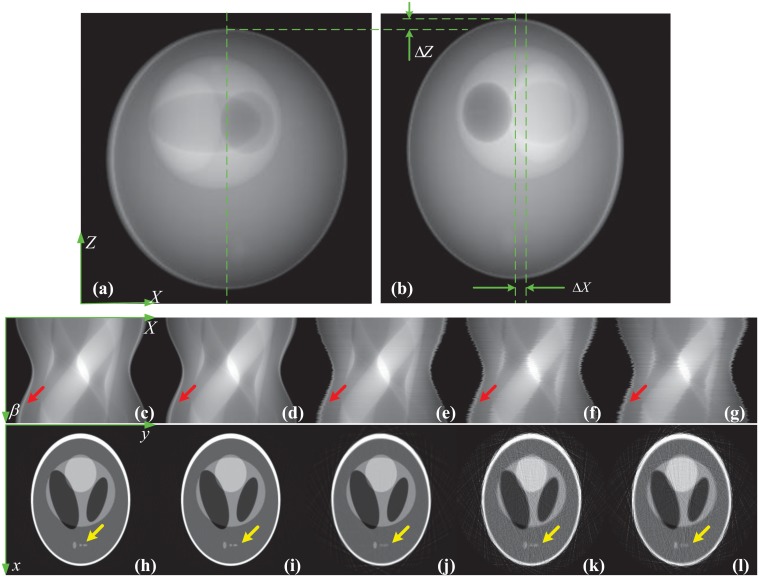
The influence of the geometry misalignment on the CT images. (a) and (b) are two projections under two different view angles. (c)-(g) are the projection sinograms and (h)-(l) the reconstructed CT images. (c) and (h) correspond to the case without jittering, (d) and (i) with shift along axis *x*, (e) and (j) with shift along axis *y*, (f) and (k) with shift along axis *z* and (g) and (l) with shifts along *x*, *y* and *z* simultaneously.
